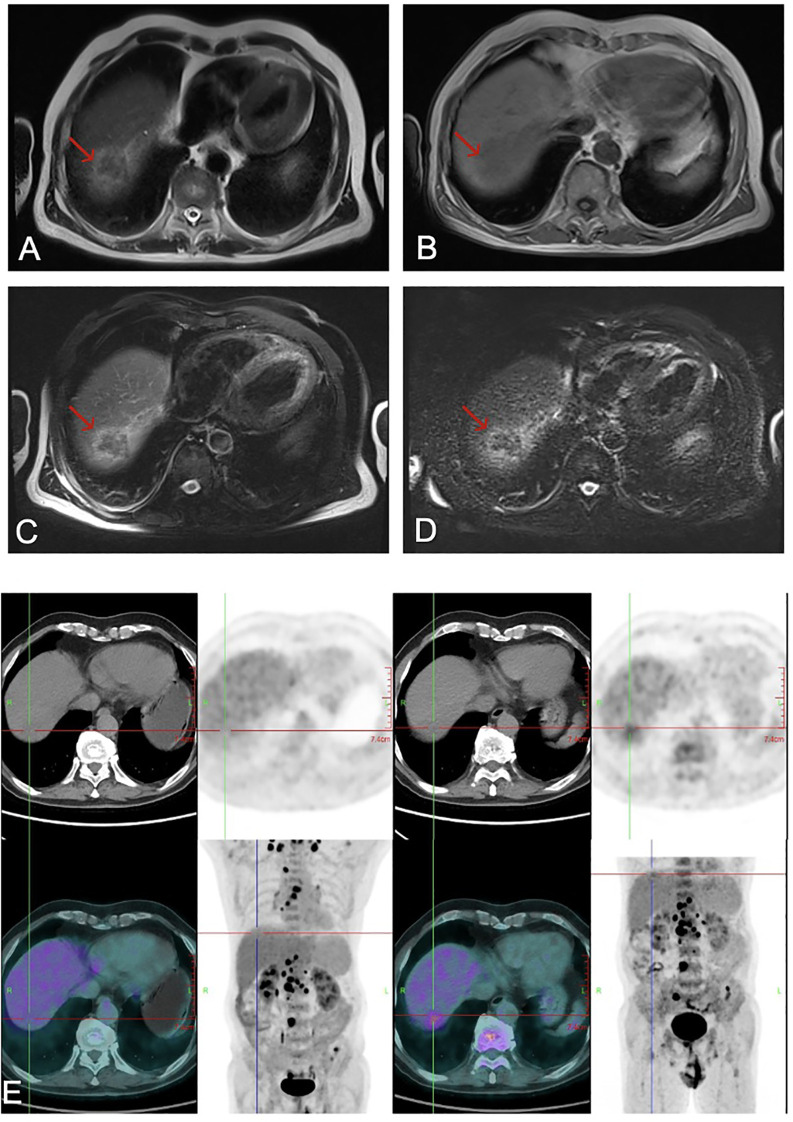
Abdominal MRI revealed a tumor (red arrow) in the right liver: (A) T2-weighted image; (B) T1-weighted image; (C) T2-weighted fat-suppressed image; and (D) diffusion-weighted image (DWI); (E) 18F-FDG PET-CT demonstrated widespread hypermetabolic foci throughout the body, consistent with disseminated metastatic disease. 18F-FDG, 18F-fluorodeoxyglucose; PET-CT, positron emission tomography–computed tomography; MRI, magnetic resonance imaging.
